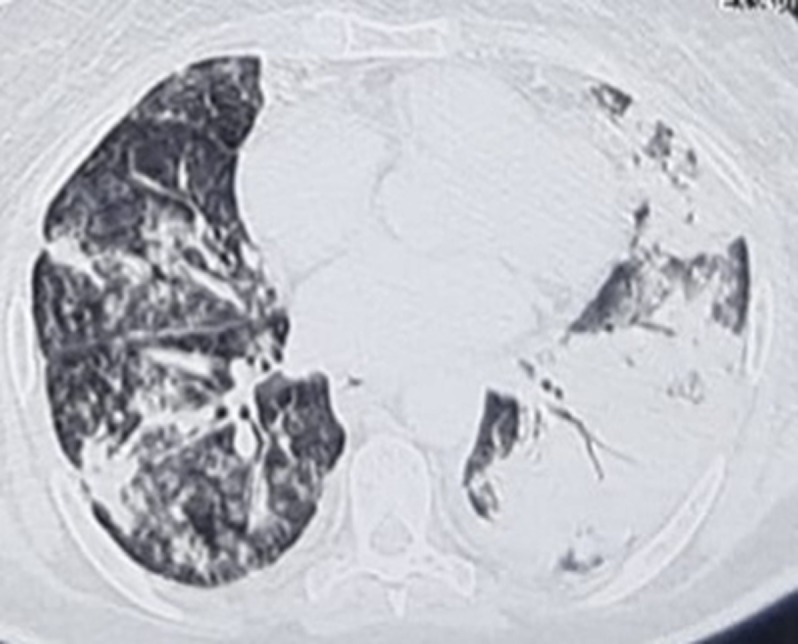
thoracic CT scan showing bilateral extended alveolar interstitial infiltrates exceeding 75% in the left side in favor of COVID-19
